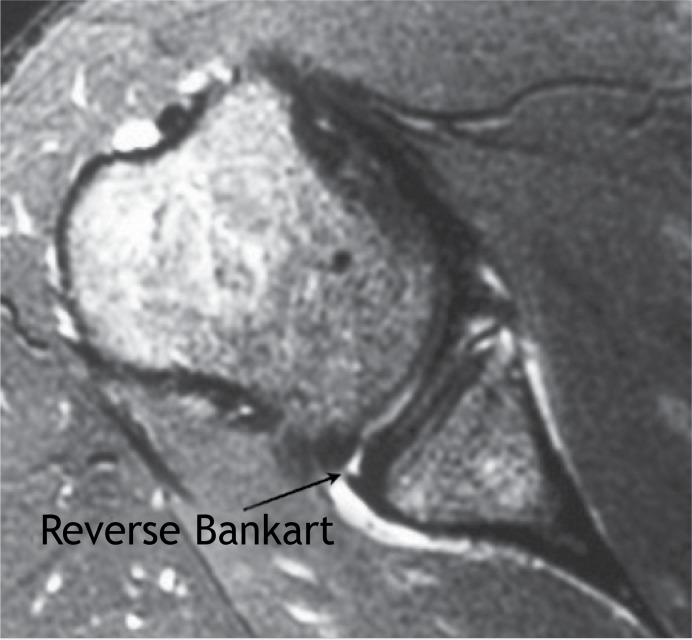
Magnetic Resonance Image of posterior labral injury.
